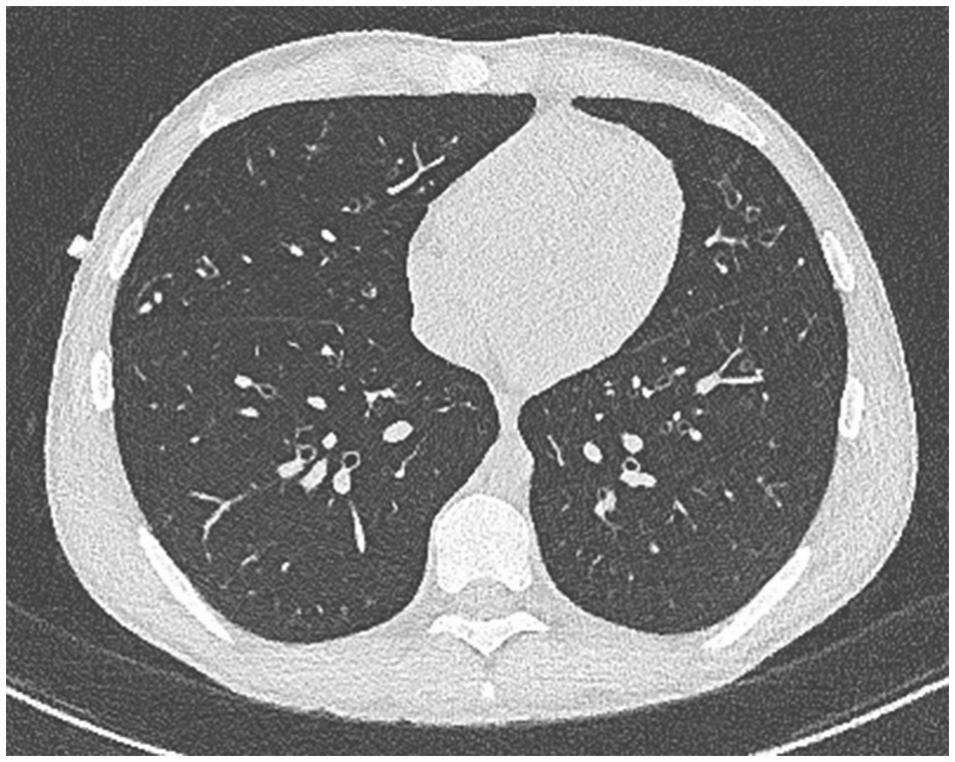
High resolution CT chest with contrast. Generalized bronchiectasis demonstrated.
